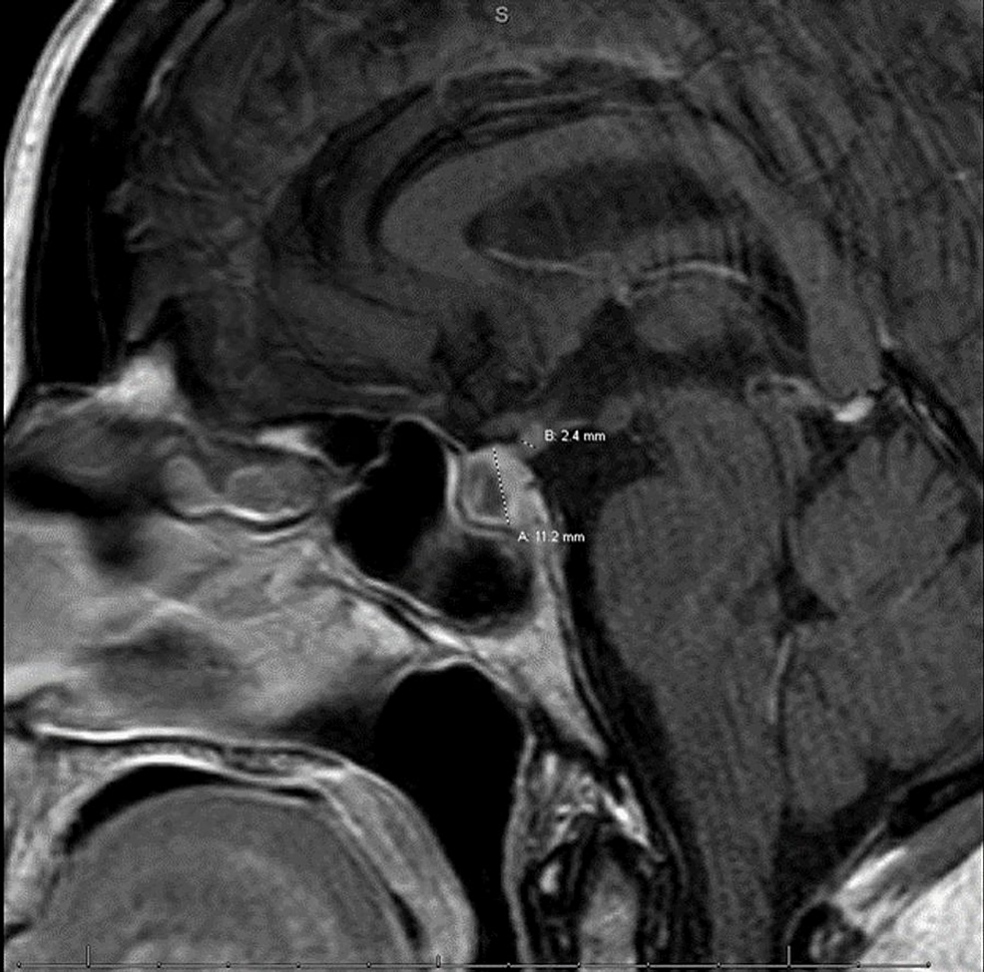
MRI of the brain with contrast T1 weighted sagittal cross section highlighting abnormal enhancement and thickening demonstrating inflammation of the pituitary gland and stalk
